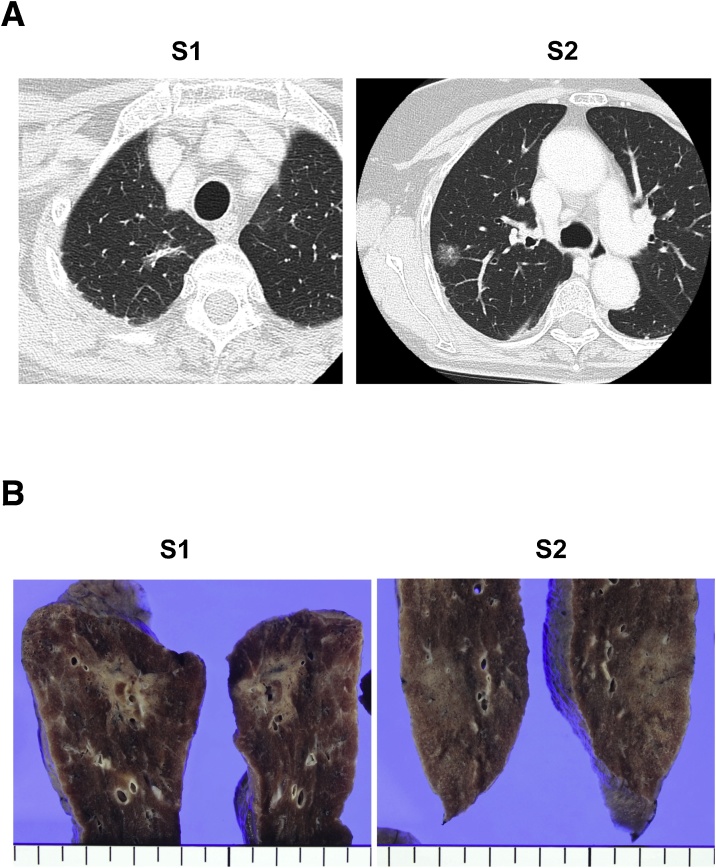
A. A computed tomography of the chest showing a band-like shadow with ground-glass opacity (GGO) in the apical segment (S1) of the right upper lobe of the lung. A GGO that contains a solid component was also detected in the posterior segment (S2). Both these two regions were considered as early stage lung cancer (S1: cT1aN0M0 Stage1A1, S2 cT1bN0M0 Stage1A2). B. Macroscopic findings of the tumor. Pathological diagnosis of the S1 region was non-invasive adenocarcinoma (pT1bN0M0, Stage1A2). The S2 region was diagnosed as invasive adenocarcinoma (pT1aN0M0, Stage1A1).
